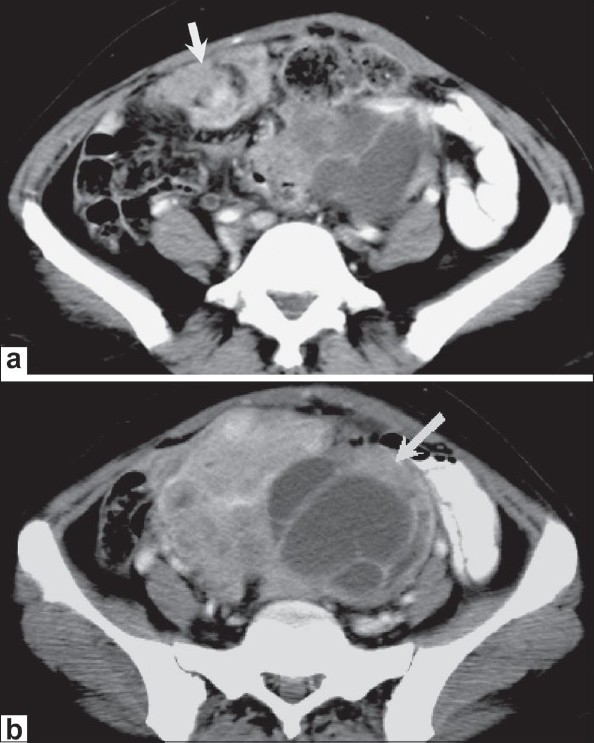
Peritoneal carcinomatosis. Axial contrast-enhanced CT scan of the mid-pelvis (a) shows a heterogeneously enhancing peritoneal/omental deposit (arrow). An axial image (b), a little inferior to A shows a large heterogeneous mass (arrow) in the pelvis with solid and cystic components. Biopsy showed papillary adenocarcinoma of the ovary
